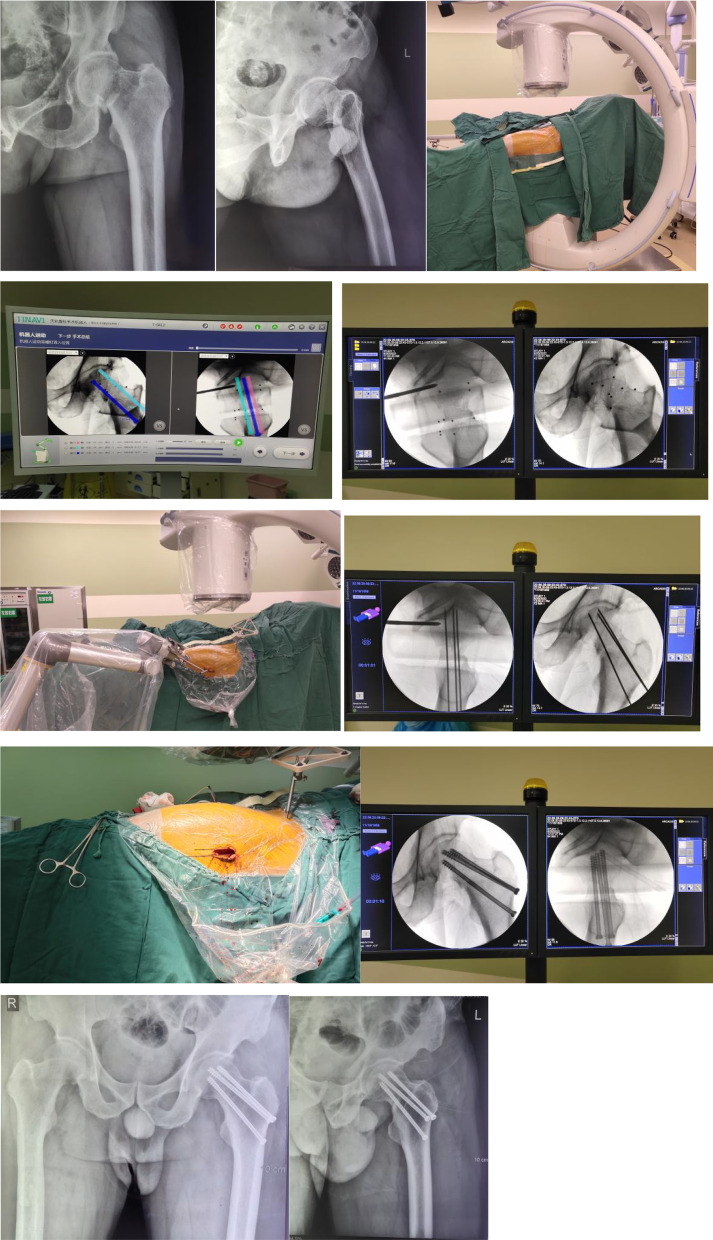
Patient 1, male, 56 years old, left femoral neck fracture (a, b) X-ray examination shows: left femoral neck fracture, Garden type III. c After traction on the affected limb and satisfactory alignment of the femoral neck fracture, routine lower limb disinfection was performed. d, e Computerized planning of the femoral neck hollow nail placement path, length and diameter by the robot. f–h Use the surgical robot to position the guide cannula in the desired position, then drive three parallel guide pins in turn, fluoroscopy the guide pin position, direction, and length as needed. i The hollow drill bit is drilled through the lateral femoral bone cortex with the guide pins, the 7.3 mm diameter hollow screw is selected for placement with the guide pins, and the screw is tightened with gradual pressure. j, k Postoperative X-ray fluoroscopy showed satisfactory fracture repositioning and hollow screw position
